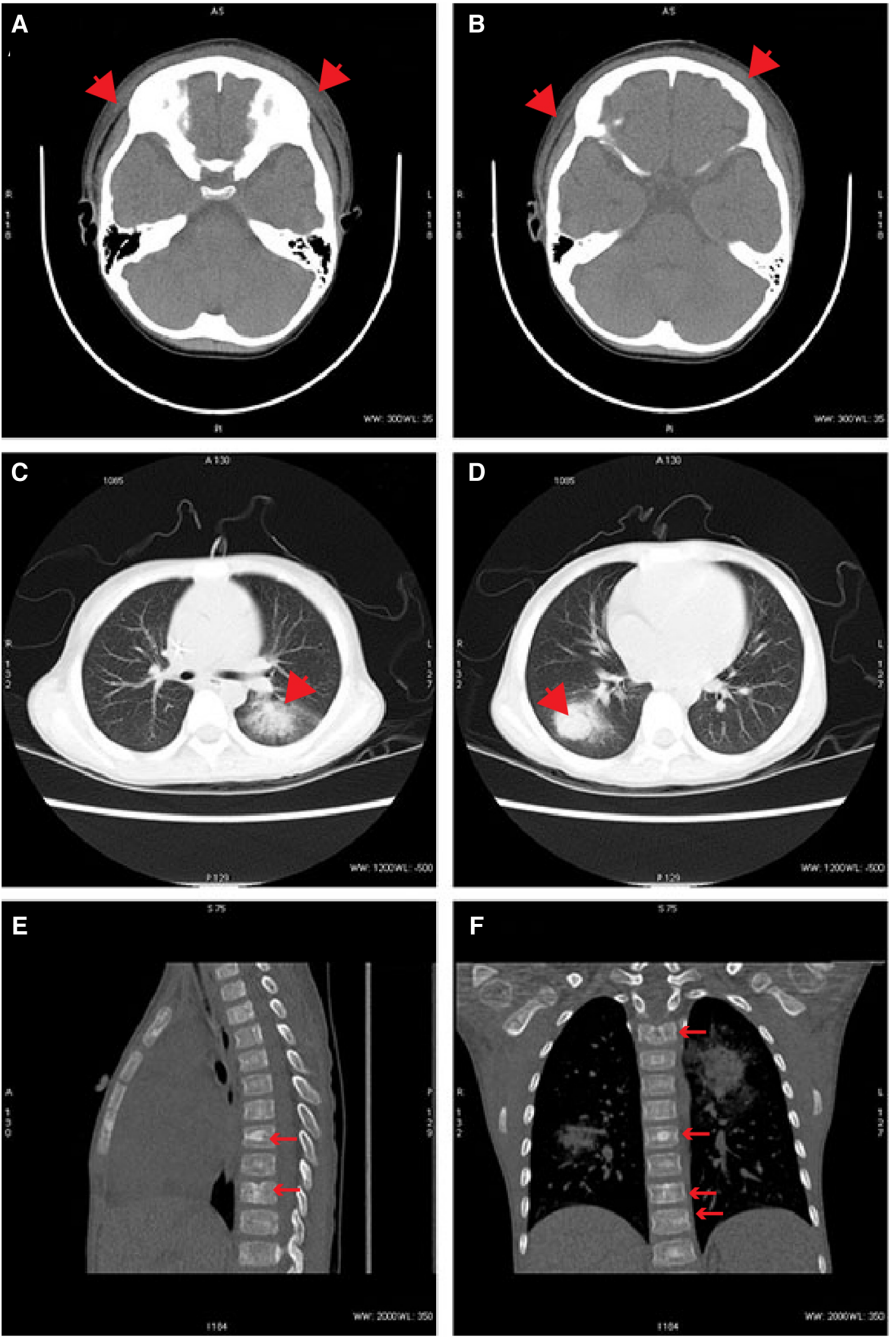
Representative radiographs. (A,B) CT images demonstrating scalp abscess on DAO 14. (C,D) CT images demonstrating pulmonary infarctions on DAO 14. (E,F) CT images demonstrating evidence of osteomyelitis on DAO 14.
